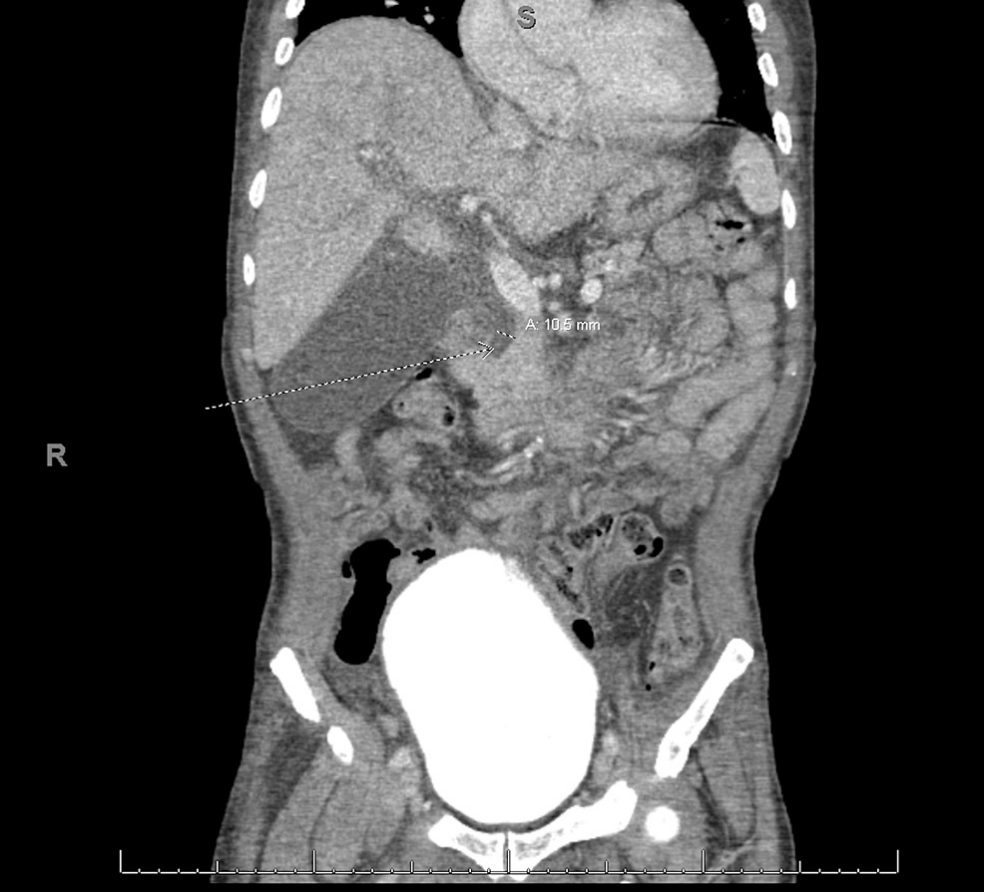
CT showing distal bile duct stones and extrahepatic ductal dilation (10.5 mm) CT: computed tomography
